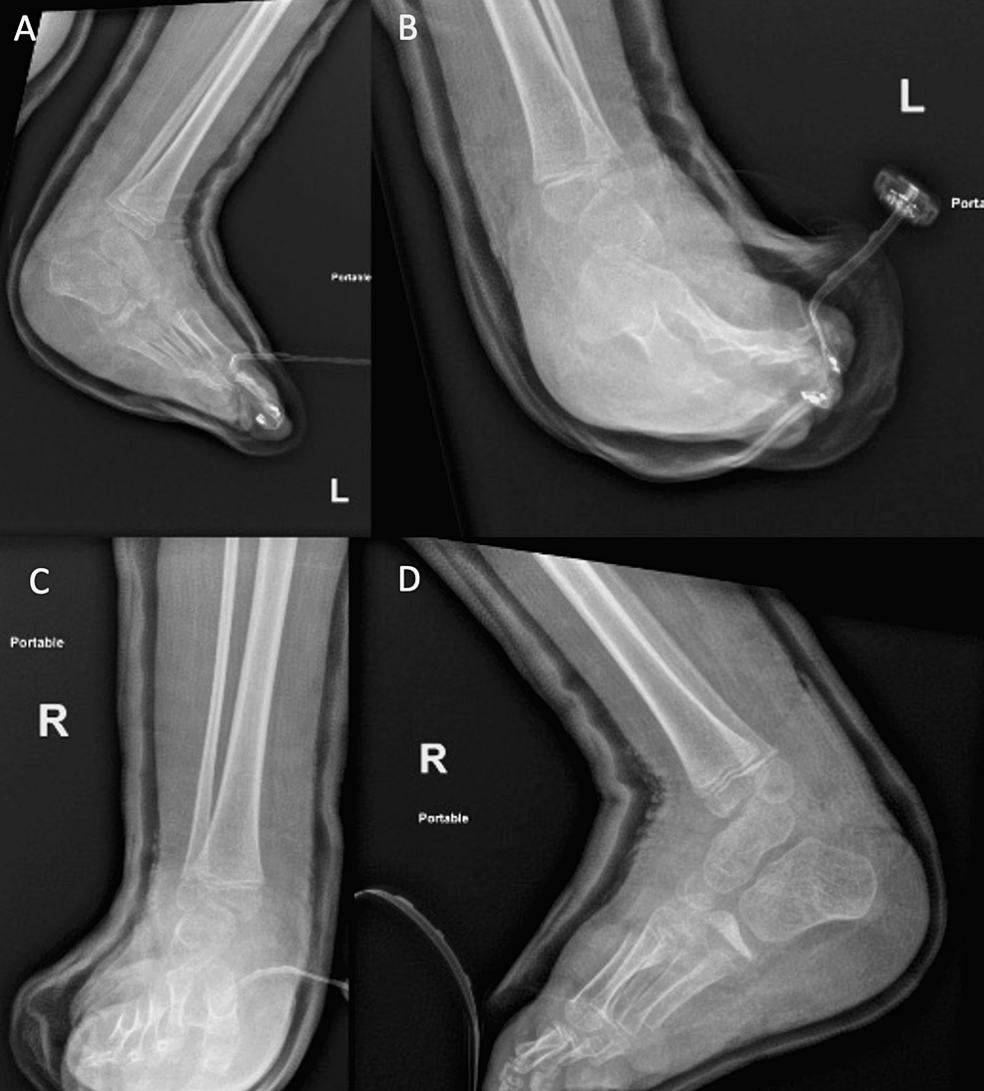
Immediate postoperative x-ray images of Case 2 (A) Lateral x-ray image of the left foot. (B) Anteroposterior (AP) x-ray image of the left foot. (C) AP x-ray image of the right foot. (D) Lateral x-ray image of the right foot.
